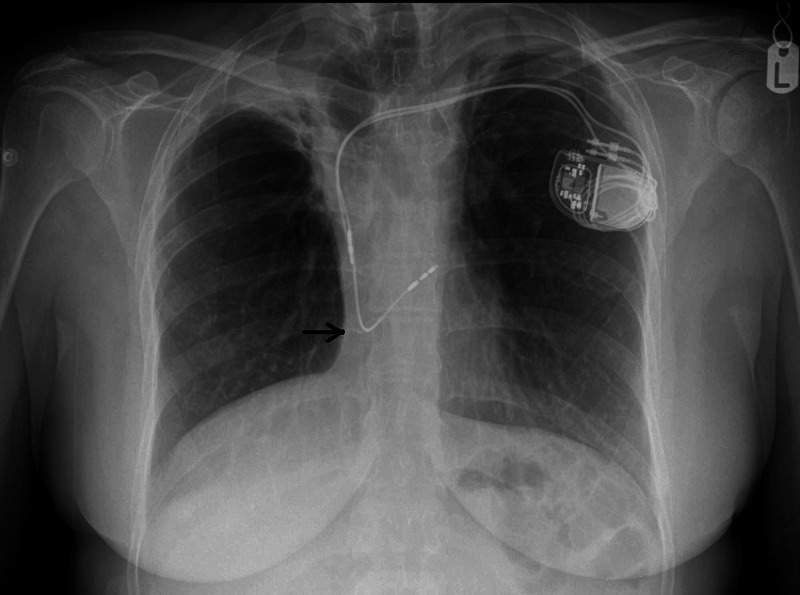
Post permanent pacemaker implantation, chest X-ray shows active leads fixed to the right atrial appendage and right ventricular apex (arrow)
